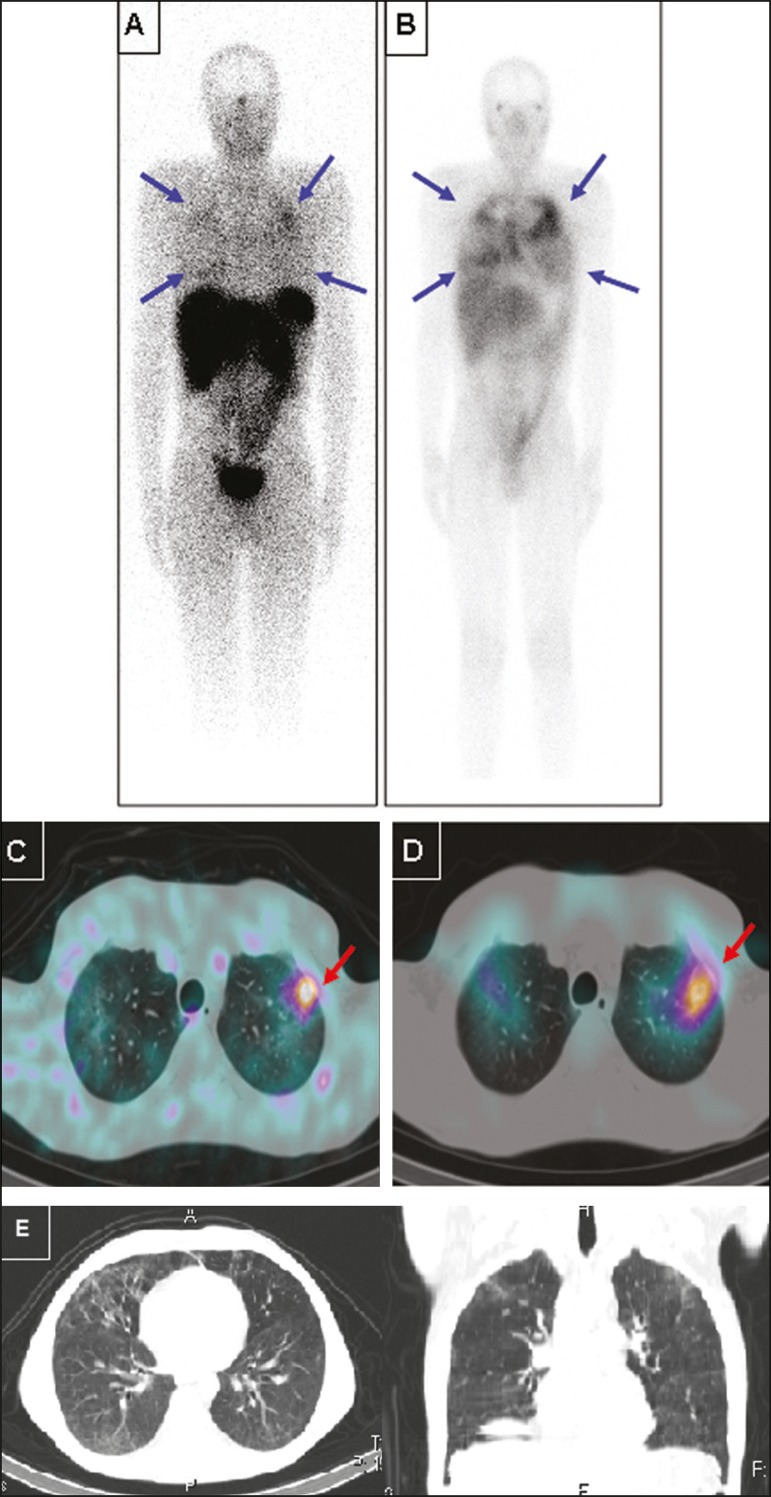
Anterior whole-body images (A,B) and transaxial SPECT/CT images (C,D)
